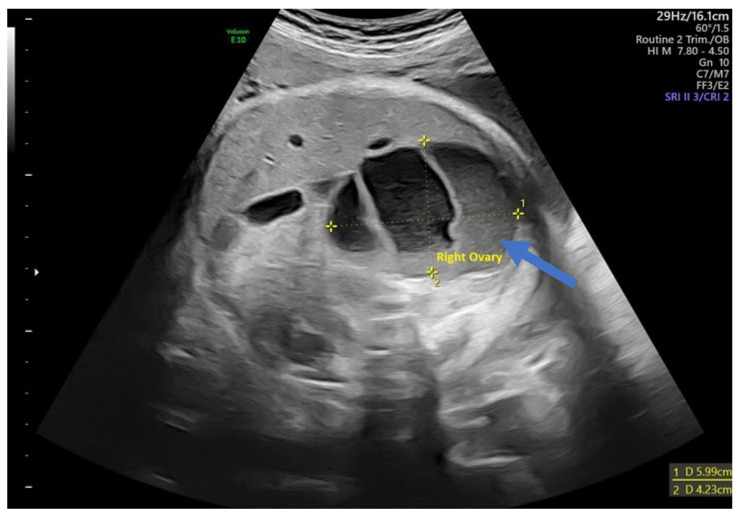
Ultrasound axial view. Right ovarian complex cyst in the 32nd week of pregnancy with a size of 6/4 cm and an echolucent stratification (arrow) corresponding to a hemorrhagic ovarian cyst.
